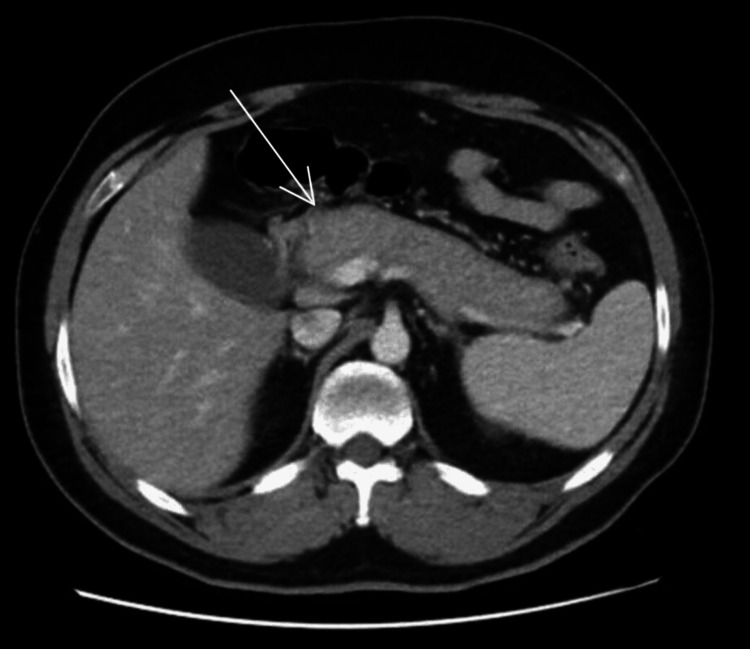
CT scan showing diffusely enlarged pancreas
